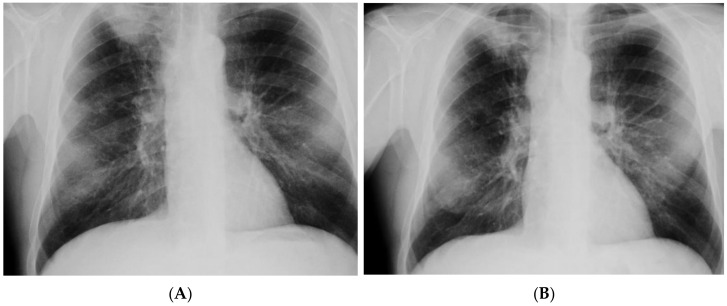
(A)—X-ray of the chest taken on 29 July 2021 (after 2 months of treatment)—irregular infiltration in the upper right field; (B)—12 October 2021 (after 5 months of treatment)—reduced infiltration.
